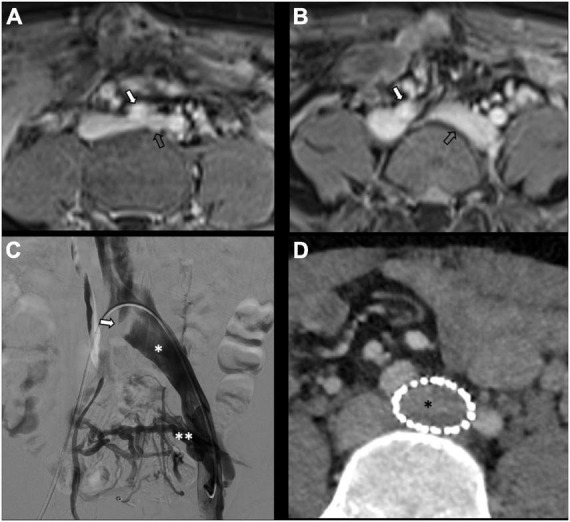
Type IIa pelvic varices in a 30 yo male due to May-Thurner syndrome. (A,B) MR phlebography shows left common internal iliac vein (open arrow) compressed by the right common iliac artery (white arrow). (C,D) Conventional and computed tomography (CT) phlebography show dilated left common iliac vein (single white asterisk) with left-sided pelvis varices (double white asterisks), treated with stenting (single black asterisk).
